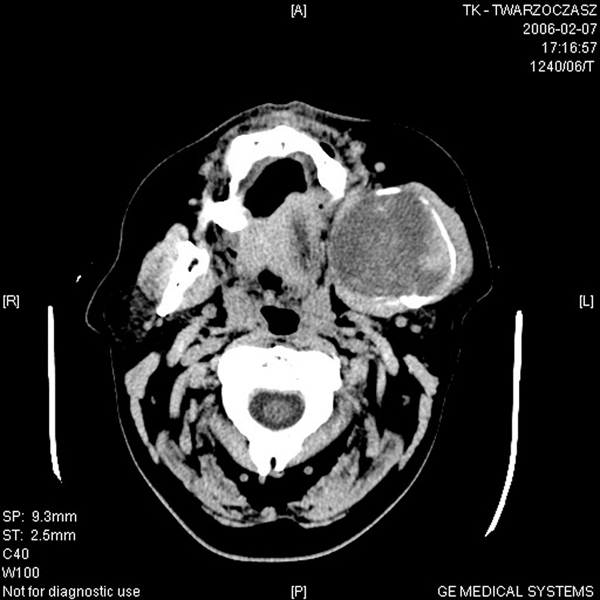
Computed tomography scan. The figure demonstrates a tumorous lesion in the area of the mandibular angle and ramus, slightly and heterogeneously enhanced after an intravenous contrast agent.
